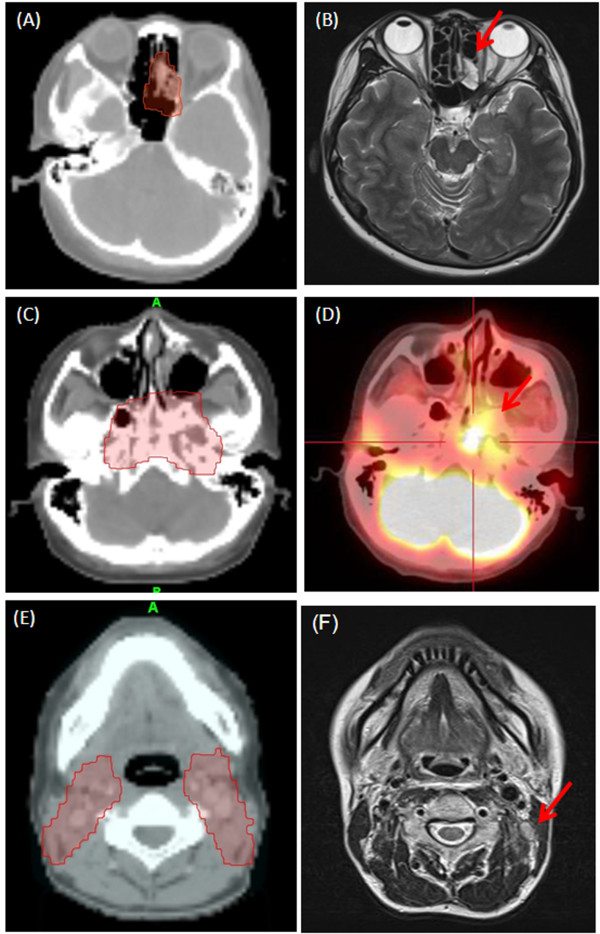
The comparison of original planning dose distribution (red color area) and locoregional failure (red arrow). For patient 1. (A) In 2007/4, planning Dose distribution of 70 Gy (red color area) at ethmoid sinus; (B) In 2008/1, MRI images show a local relapse. For patient 2. (C) In 2008/11, planning dose distributions of 71.6 Gy (red color area) at skull base; (D) In 2009/3, PET-CT images show a at skull base, SUV_max _= 6.4. For patient 3. (E) In 2008/7, planning Dose distributions of 70 Gy (red color area) at neck lymph node and lymphatic drainage; (F) In 2009/6, MRI images show a regional lymph node relapse.
